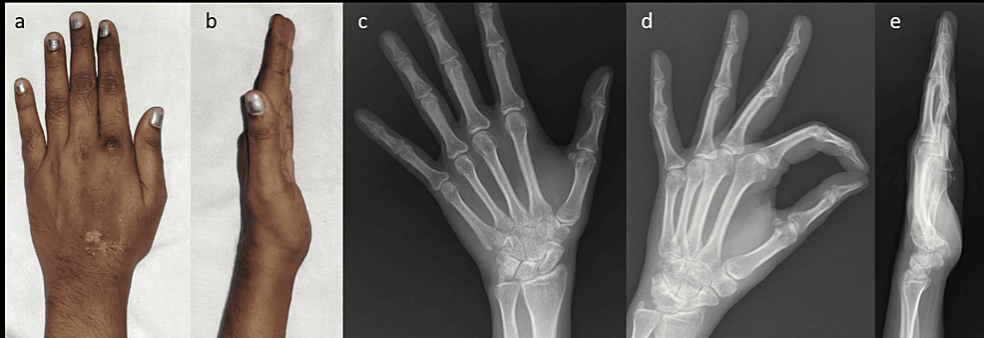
Clinical pictures and X-rays after K-wire removal Clinical pictures post-K-wire removal showing (a) dorsum and (b) lateral aspect of hand, (c) anteroposterior (d) oblique and (e) lateral views after K-wire removal
